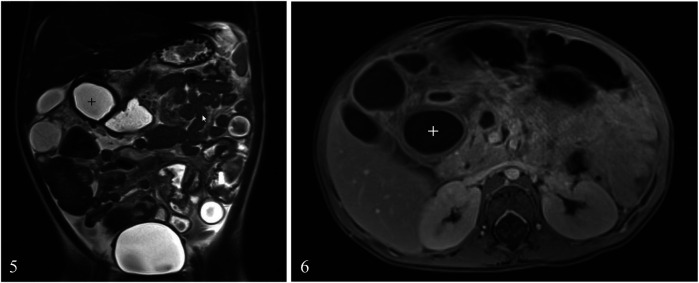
MRI scan showing double-walled cyst (+).
